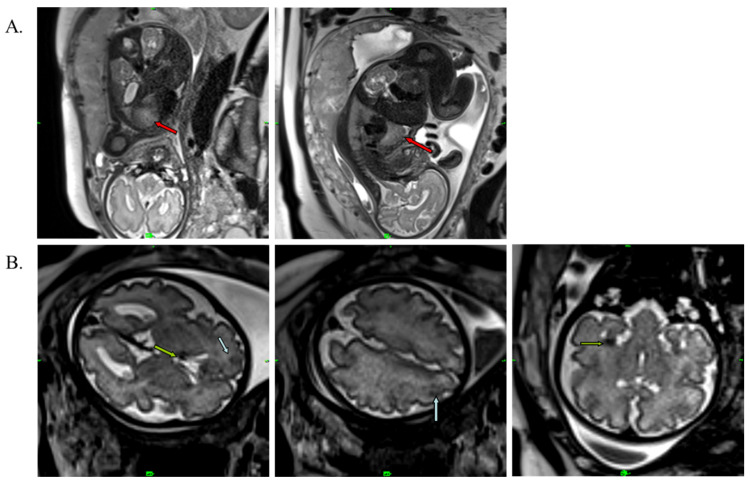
T2-weighted MRI image of the fetus at the 30th week of gestation. (A) Red arrows show cardiac rhabdomyoma (31 × 32 × 33 mm). (B) Blue arrows show cortical tubers, and green arrows represent subependymal nodules in the brain.
